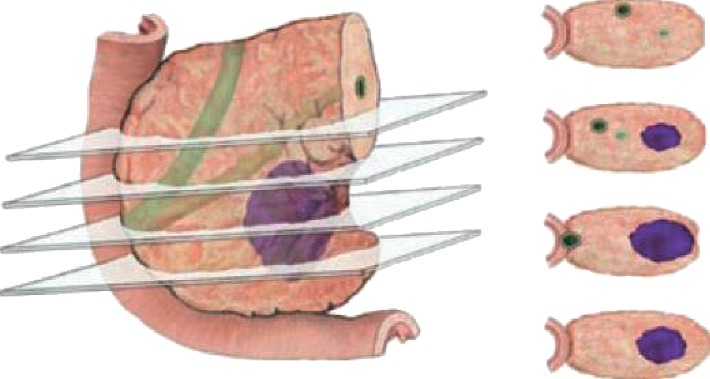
Choose three CT slices [11].
